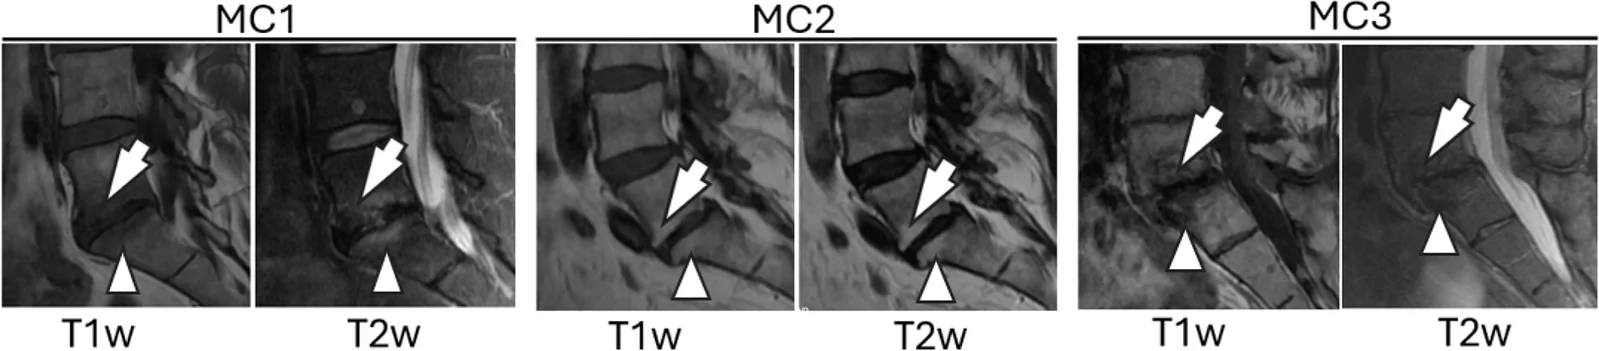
Representative T1- and T2-weighted MRI images of Modic type 1, 2 and 3 changes. MC1, Modic type 1 changes; MC2, Modic type 2 changes; MC3, Modic type 3 changes.
